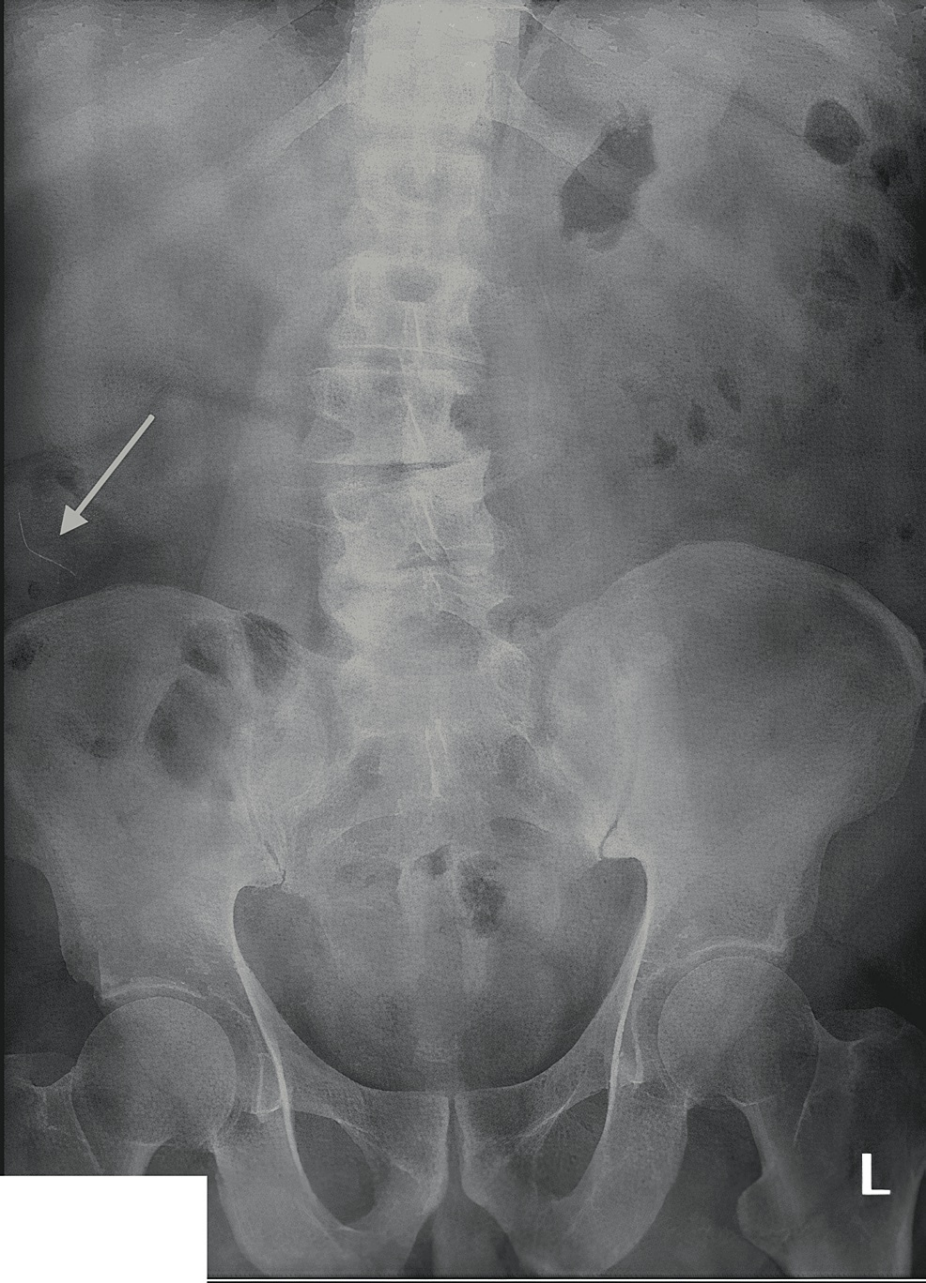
Pre-operative abdominal radiograph demonstrating a curvilinear density within the right lower quadrant (white arrow noting radiopaque density)
